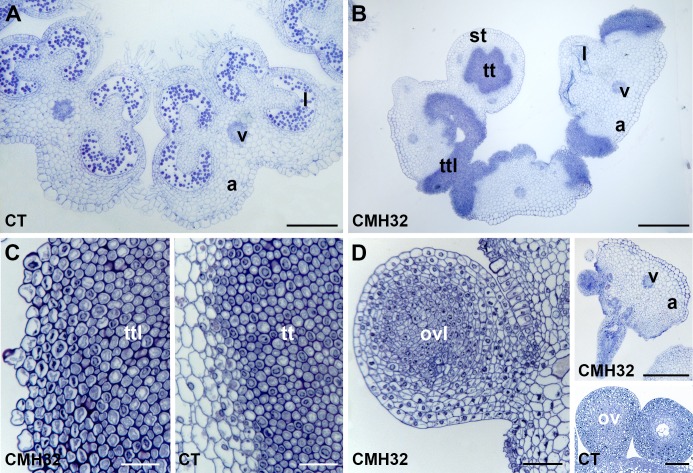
Cross sections of anthers and pistils from mature flowers of the tomato cultivar Red Setter grown under control and continuous mild heat conditions. A, overview of anthers grown under control conditions (CT). Scale bar: 300 μm. B, overview of anthers and pistil from CHM32. Scale bar 300 μm. C, transmitting-tissue-like cells from an anther grown under CMH32 (close up of B) on the left, and true stylar transmitting tissue from control conditions on the right. Scale bar 20 μm. D, ovule-like deformation from an anther grown under CMH32 (scale bar 30 μm). Upper inset shows overview of CMH32 anther with ovule-like deformation (scale bar 300 μm), lower inset shows a true ovule from control conditions (scale bar 50 μm). a, anther; l, locule; ov, ovule; ovl, ovule-like structure; st, style; tt, transmitting tissue; ttl, transmitting tissue-like cells; v, vascular bundle.
